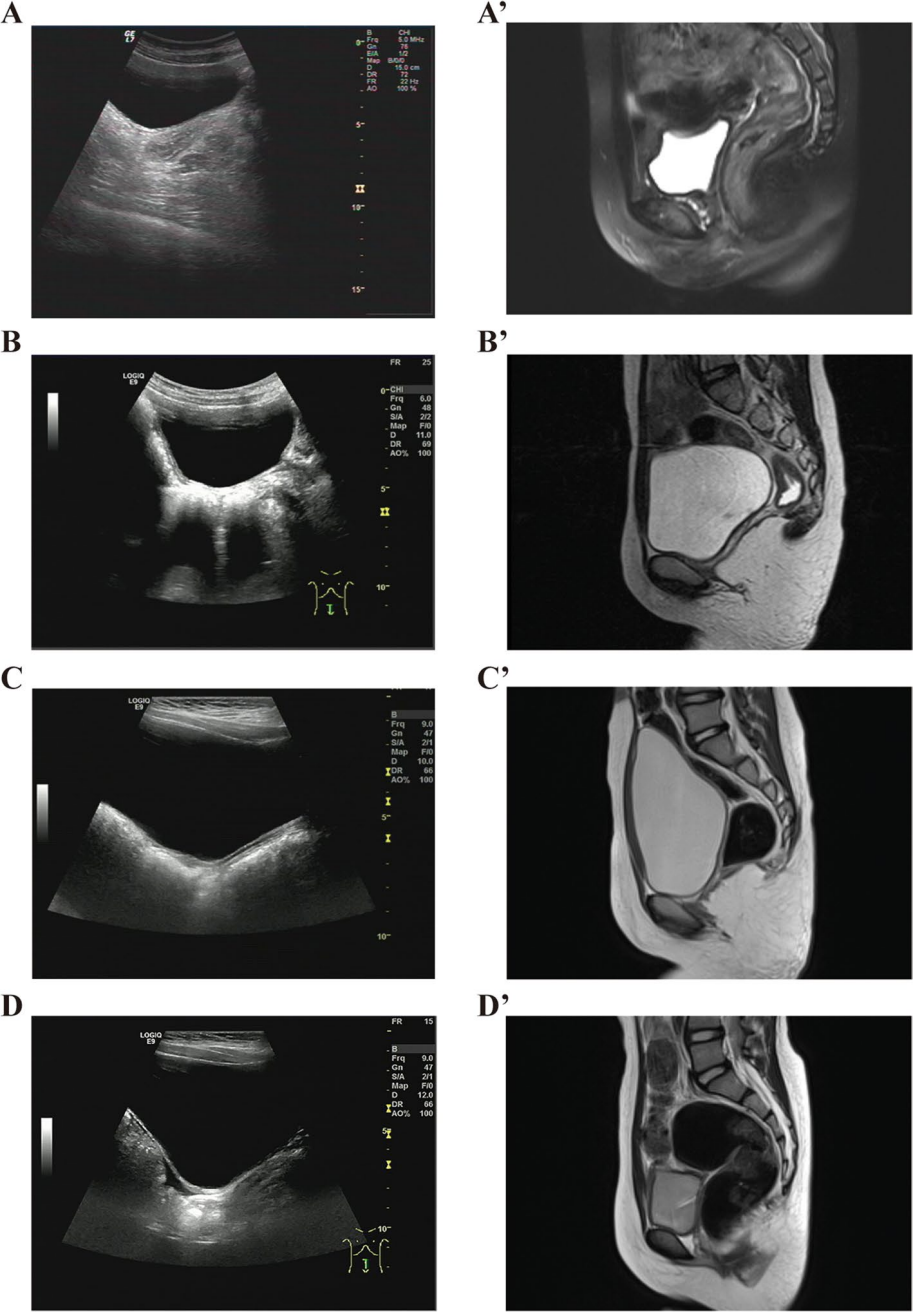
The pelvic ultrasound and MRI of case 1-case 4. A The pelvic ultrasound of case 1. A’ The pelvic MRI of case 1. B The pelvic ultrasound of case 2. B’ The pelvic MRI of case 2. C The pelvic ultrasound of case 3. C’ The pelvic MRI of case 3. D The pelvic ultrasound of case 4. D’ The pelvic MRI of case 4
